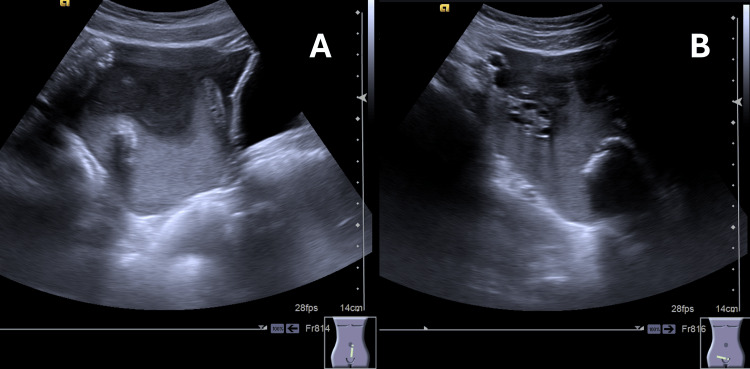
Abdominal ultrasound revealing significant free hematic peritoneal effusion
